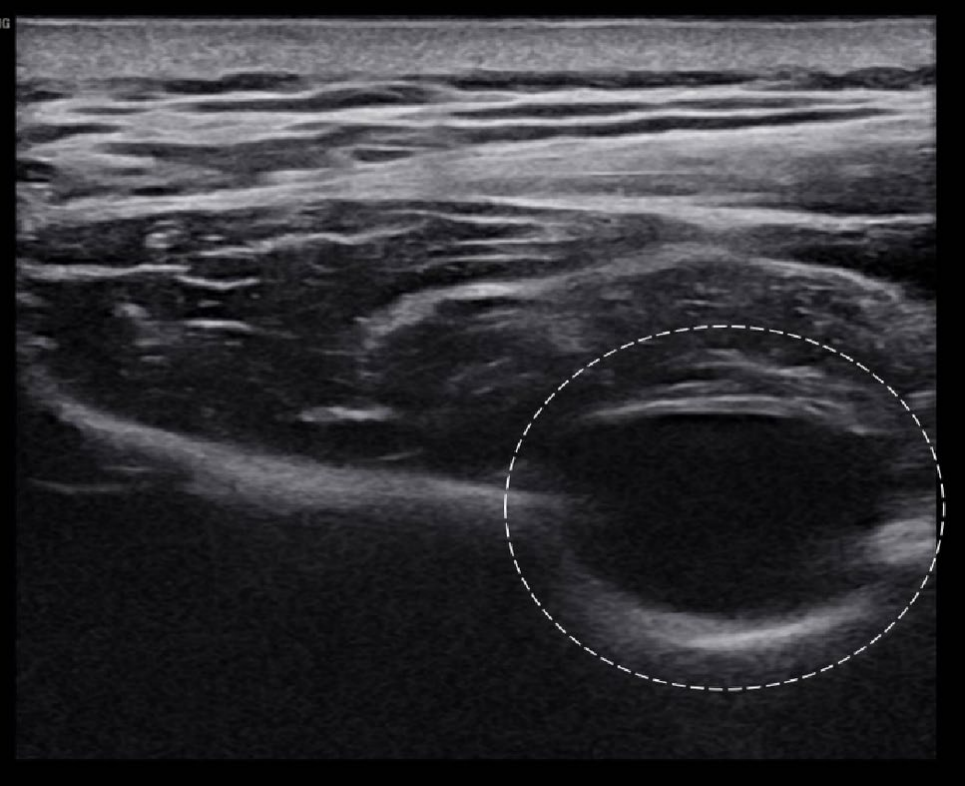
Ultrasound image showing a hypoechoic cyst near the suprascapular notch. (white circle)
